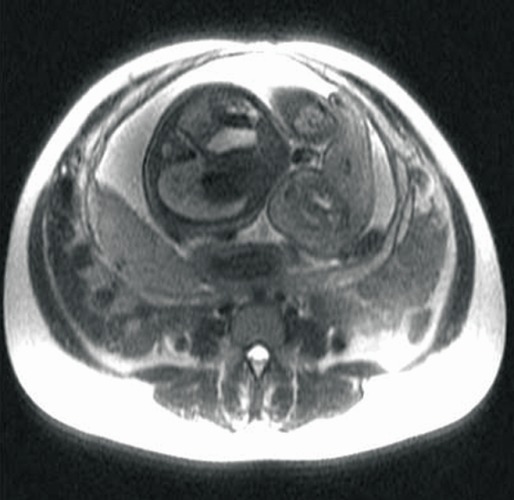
Fetal MRI–gestational age 22 weeks, viable fetus and parasite fetus (transverse plan)
